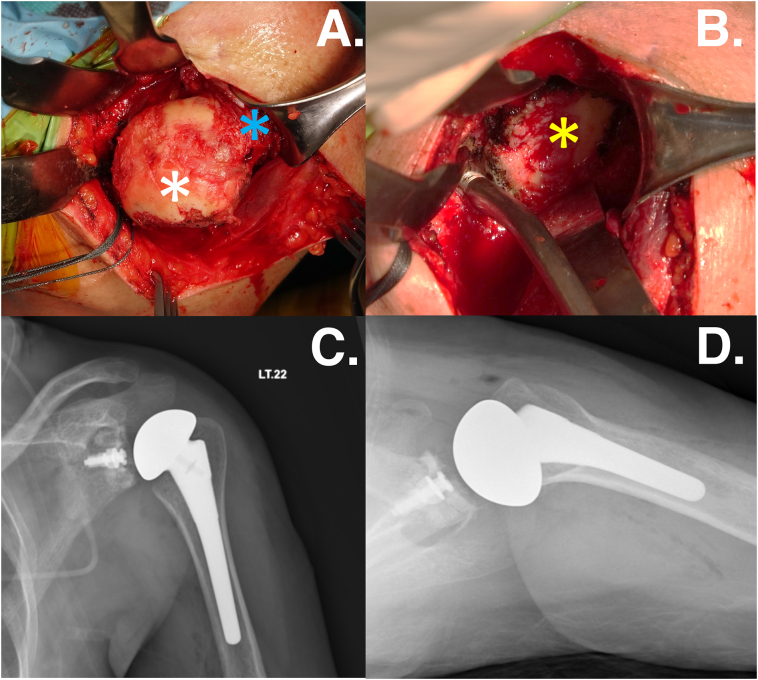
The intraoperative of the left total shoulder arthroplasty showed a.) the severe cartilage loss of the humeral head (white asterisk) and the rotator cuff was intact (blue asterisk). b.) the severe cartilage loss of the glenoid was exposed (yellow asterisk). The postoperative x-ray after performing the cementless total shoulder arthroplasty at the left shoulder. c.) the anteroposterior view and d.) the trans axillary view. (For interpretation of the references to colour in this figure legend, the reader is referred to the web version of this article.)
